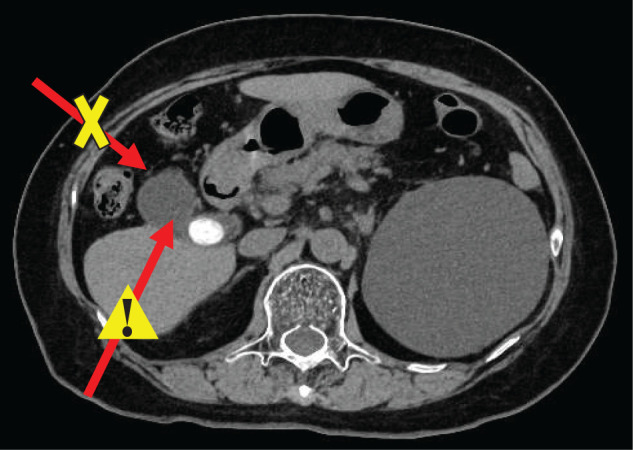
Abdominal CT findings. Abdominal CT showing hypoplasia of hepatic segment 4 and gallbladder distension with impacted gallstones. The arrows indicate the potential percutaneous puncture routes. The anterior approach (arrow with a cross) is not feasible due to bowel interposition. The posterior approach (arrow with a warning sign) is considered unsafe because of the risk of pneumothorax. These findings suggest that percutaneous gallbladder drainage is technically difficult in this case.
